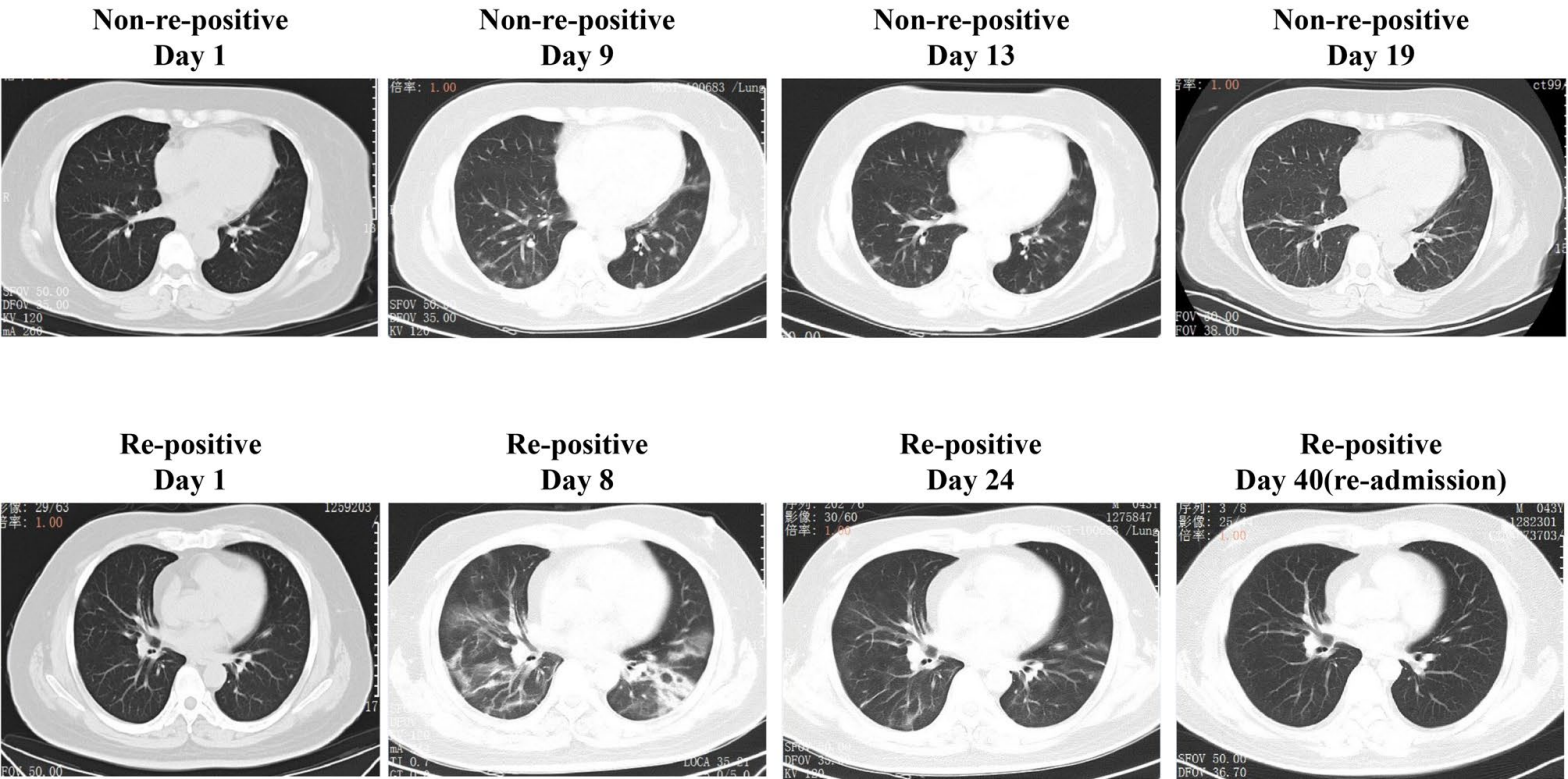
CT scan of representative patients in both non-re-positive patients and re-positive patients. Non-re-positive patients: Female, 61yo, few ground-glass opacities in bilateral lung field at day 1. Deterioration occurred subsequently (day9), and those lesions were absorbed in the following week (day 13 and day 19), accompanied by symptoms relief. Re-positive patient: male, 42yo, few ground-glass opacities in bilateral lung field at day 1. Deterioration occurred subsequently (day 8), and those lesions were absorbed in the following week (day 24), accompanied by symptoms relief. The lung lesions in CT scan were almost absorbed when found re-positive (day 40), without any recurrence of clinical symptoms.
